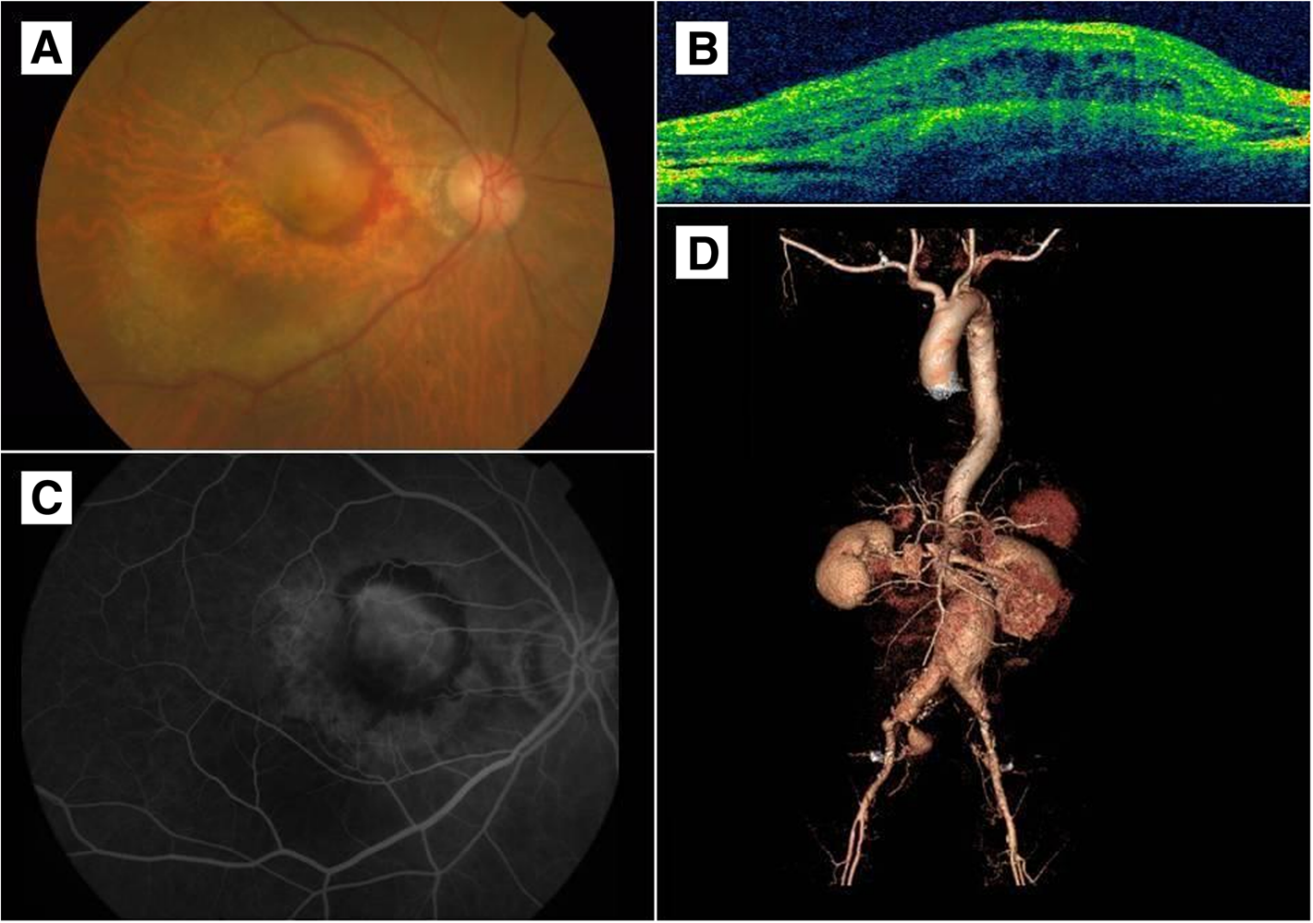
Fundus photo, optical coherence tomography, fluorescein angiography and computed topography of patient. (A) A fundus examination revealed macular elevations with subretinal neovascular membranes. (B) Optical coherence tomography showed submacular elevations with intraretinal edema. (C) Fluorescein angiography showed macular fluorescein leakage. (D) A contrast-enhanced computed tomography scan revealed an abdominal aortic aneurysm, 5.8cm in its largest diameter, with ruptured state and bilateral iliac artery aneurysms.
